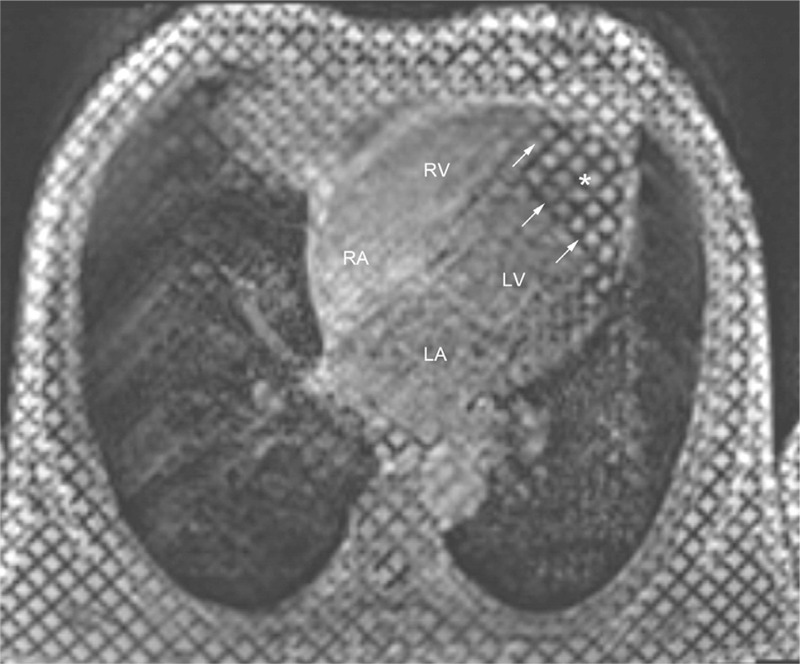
End-systolic tagging cine-MRI image in the LV 4-chamber long axis plane. Singe tagging image shows decreased myocardial systolic function in the LV apex area (lack of grid-deformation; ∗ delineates the contour of the thrombus; white arrows indicate the borders of the thrombus). LV = left ventricular, MRI = magnetic resonance imaging.
